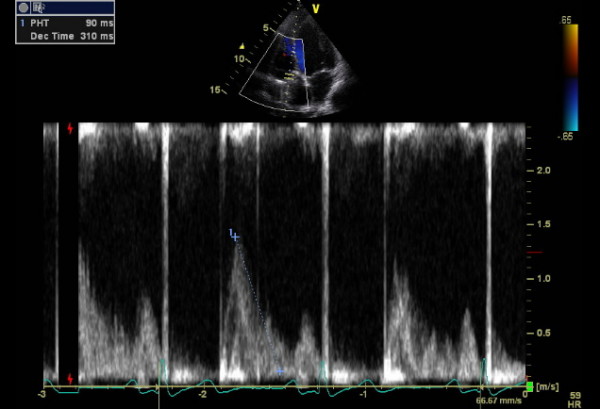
Post thrombolysis Doppler echocardiogram showing complete resolution of tricuspid prosthetic thrombus as suggested by a normal pressure half time.
